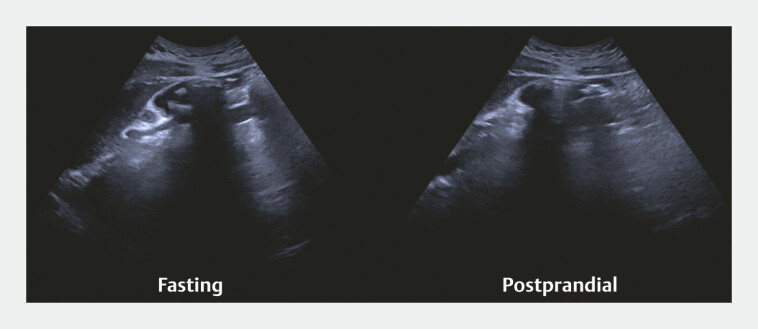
Abdominal ultrasonography indicated multiple large stones in the gallbladder and an ejection fraction of 80% on a fatty meal test.
